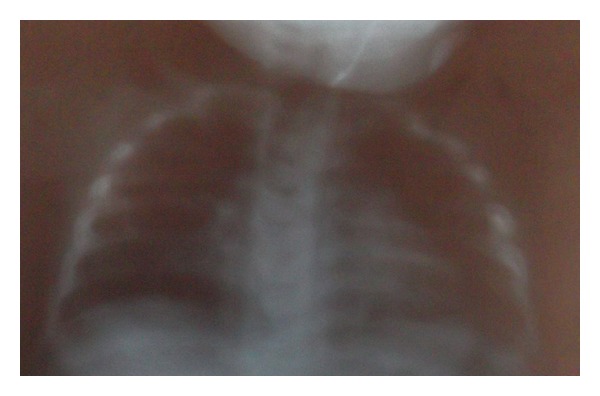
Chest Radiograph done a month after discharge.
